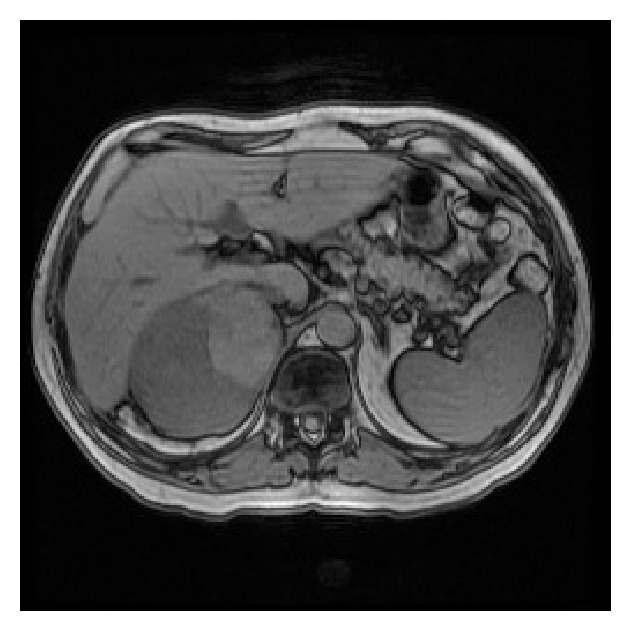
Complex right adrenal mass on MRI with no evidence of invasion.
